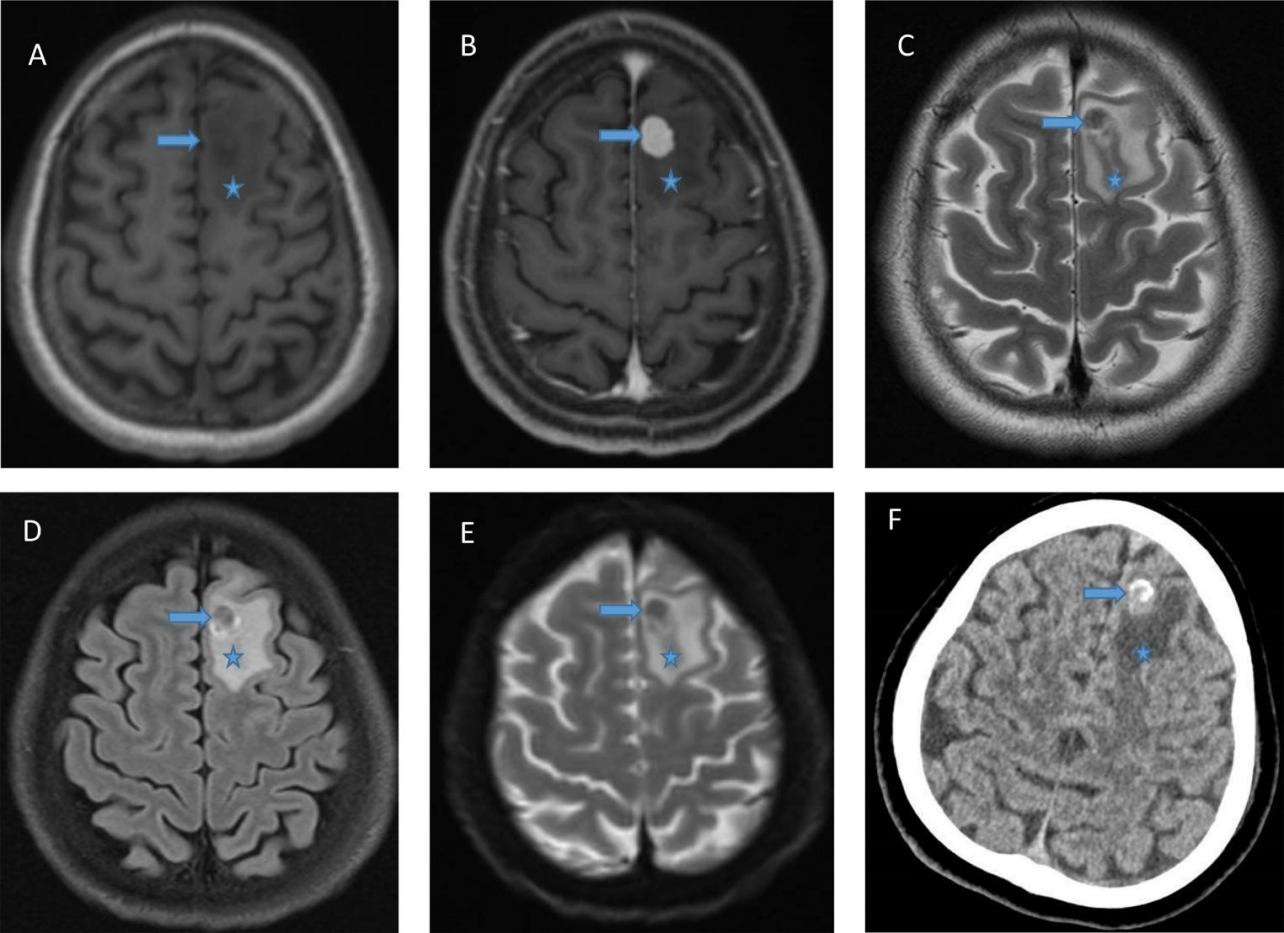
A. MRI: T1 showing circumscribed intra-axial nodule in the left
